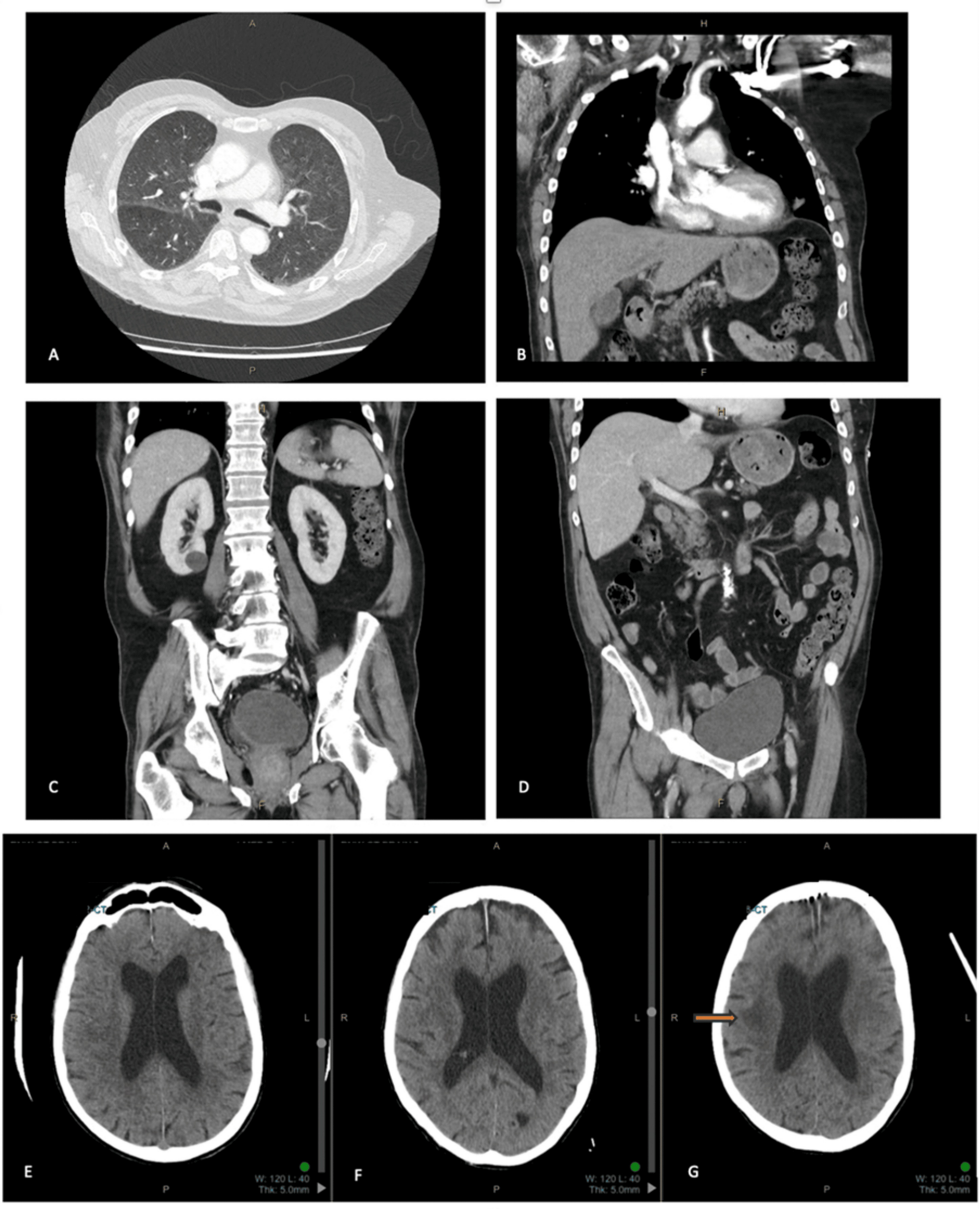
Contrast computed tomography (CT) of the chest: axial (A), coronal (B). Normal study. (C, D) Contrast CT of the abdomen and pelvis, coronal. Normal study. Non-contrast CT of the brain: day 1 (E), day 3 (F), day 4 (G). Disease progression with developing right-sided periventricular hypodensities (arrow)
